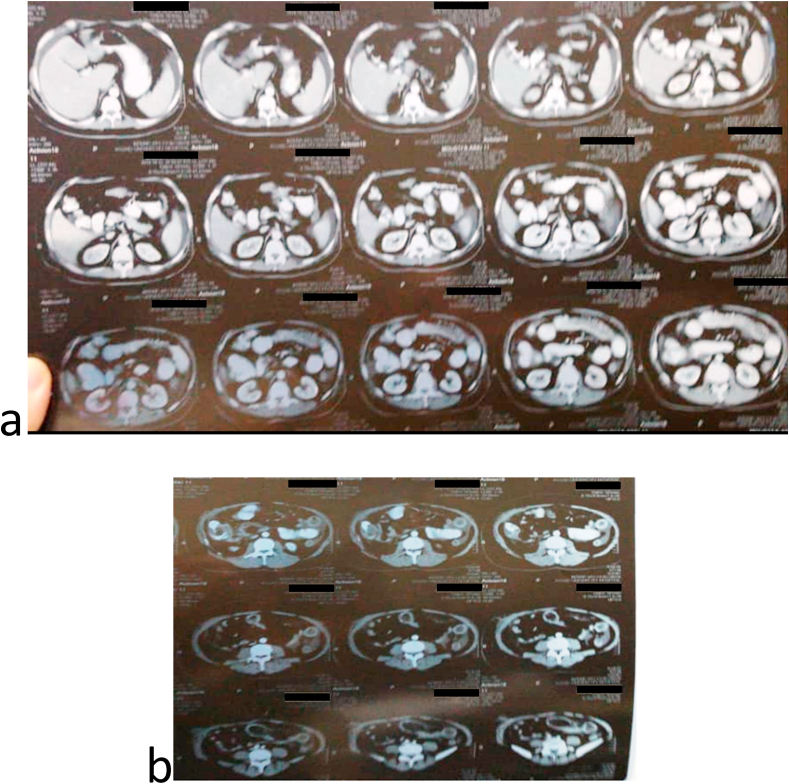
Contrast abdominal computed tomography scan: a) A fistula between the 2nd segment of duodenum and cecum, cecal wall thickening, mild enlarged pericecal lymph nodes, b) small bowel wall thickening in the left upper quadrant and change in the density of the fat around.
